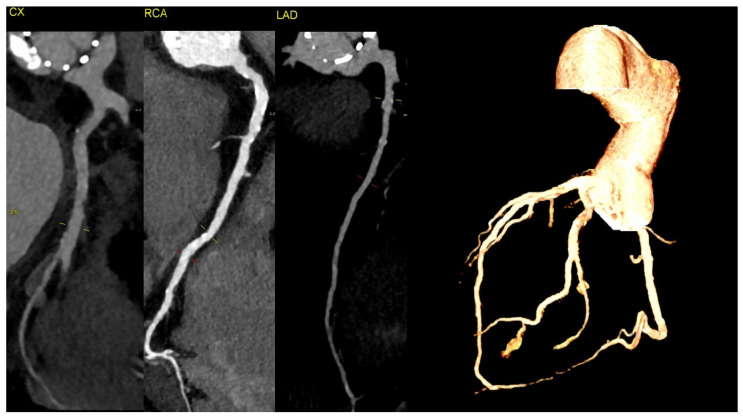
The CT discluded significant coronary artery stenosis (Cx—circumflex artery, RCA—right coronary artery, LAD—left anterior descending artery). The myocardial infarction was treated conservatively with anticoagulation due to the presence of aortic graft and valve thrombosis, and it led to the resolution of the chest pain. The clinically insignificant lesions in the coronary arteries on CT were suggestive of the fact that NSTEMI could be caused by the occlusion of the ostium of the arteries by the aortic valve thrombus.
